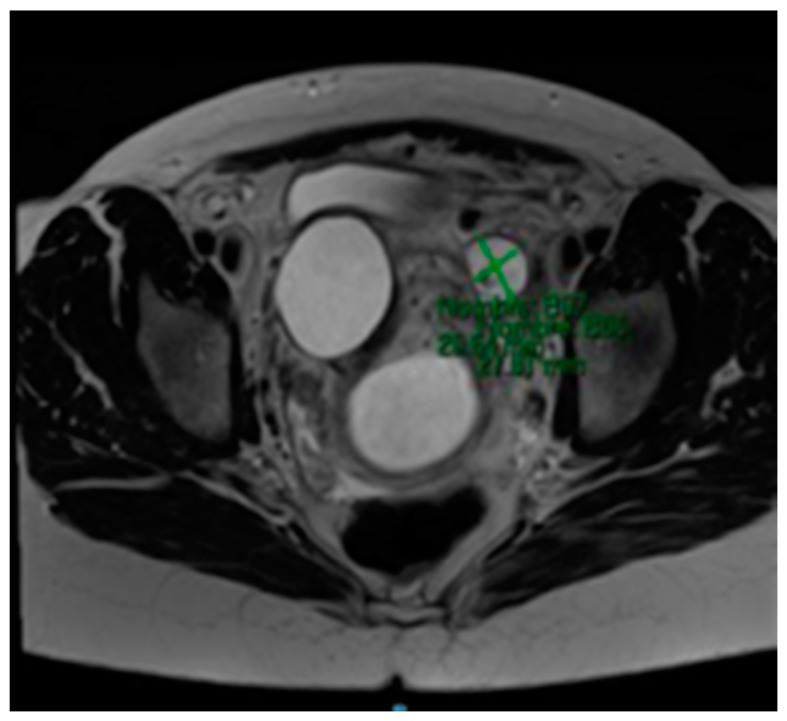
MRI scan compatible with 27 mm × 20 mm cystic formation in the left hemipelvis.
